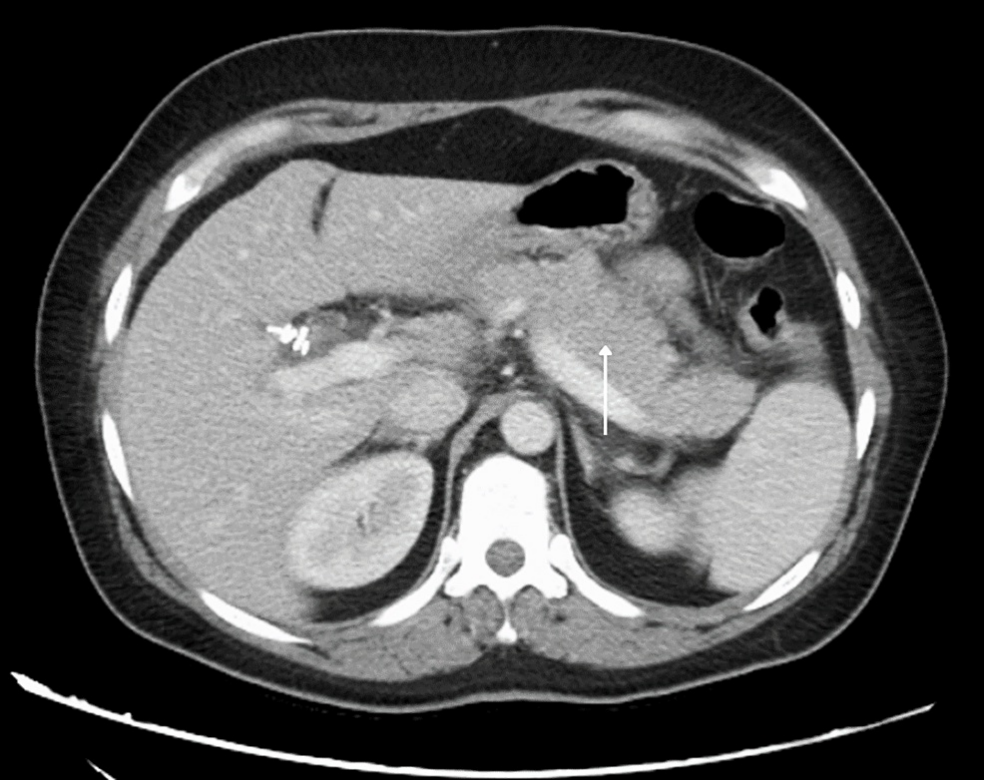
Preoperative computed tomography demonstrating an edematous, inflamed pancreas consistent with acute pancreatitis.
